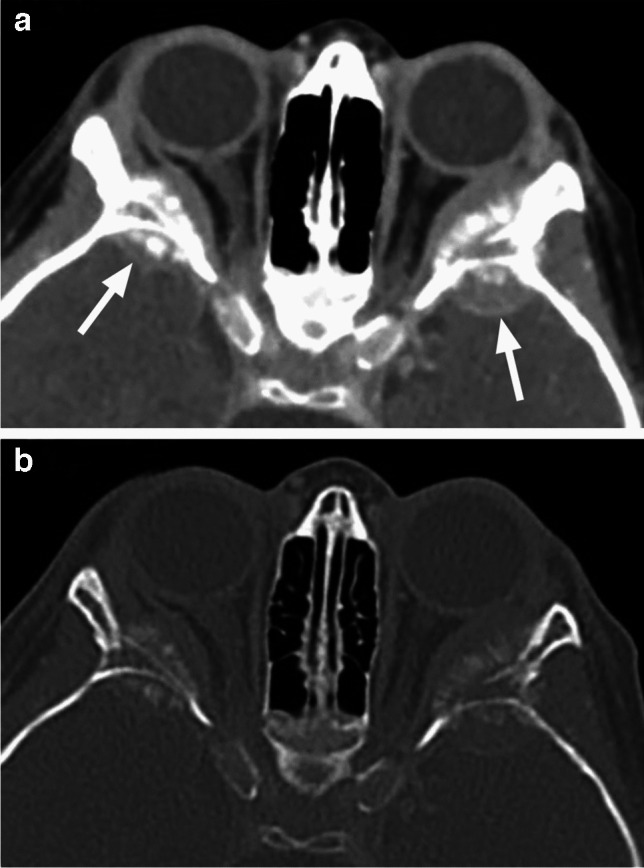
Axial postcontrast CT in soft tissue (a) and bone (b) window shows bilateral destructive lesions of the sphenoid wings/dorsolateral orbital walls with associated soft tissue components (arrows) and spiculated periosteal reaction in this patient with neuroblastoma metastases
